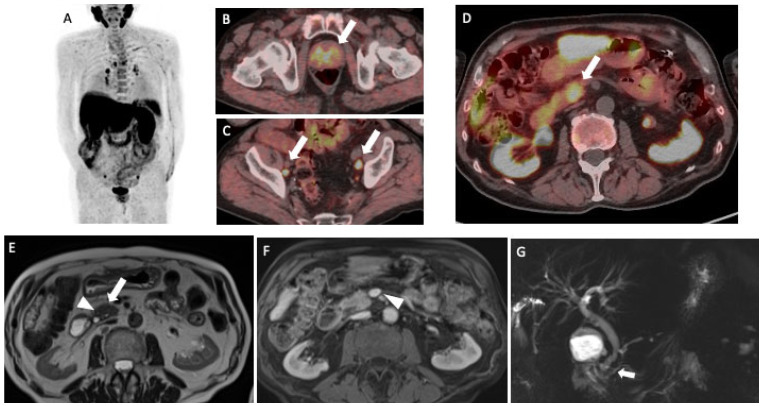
A male patient with prostate cancer underwent 18F-Choline (FCH) PET/CT for the suspicion of lymph node recurrence during hormonal therapy. He was not submitted to local therapies (i.e., surgery or radiotherapy) for the treatment of the primary prostate lesion. The patient has, in addition, a history of diabetes mellitus type II and hypertension. PET/CT detected multiple areas of focal FCH uptakes ((A); maximum intensity projection—MIP) in prostate gland (arrow, (B)) and abdominal-pelvic lymphadenopathies (arrows, (C)), compatible with recurrent prostate cancer. Moreover, a focal tracer uptake was shown in the uncinate process of the pancreas with a maximum standardized uptake value (SUVmax) equal to 13.6 (arrow, (D)). As already stated by Schillaci et al. [1], FCH may physiologically show a moderate-to-high uptake in the liver and the pancreas. However, the presence of a focal uptake should be further investigated in order to make a differential diagnosis with a malignant or benign lesion. A subsequent MRI showed no definite lesion but rather a slight “mass-like” enlargement of the pancreatic head, as visible on the axial single-shot turbo-spin echo T2-weighted image (arrow, (E)). Some micro-cystic areas were appreciated in the pancreaticoduodenal groove (arrowhead, (E)). The absence of lesions was confirmed on the post-contrast pancreatic phase (axial gradient echo volumetric fat saturated image), in which pancreatic parenchyma appears homogeneous, with no solid tissue extending towards major vascular structures (arrowhead, (F)). Of note, the cholangiopancreatography sequence showed a normal-sized but irregular main pancreatic duct, with no cephalic structures (arrow, (G)). Overall, subtle morphological changes and ductal abnormalities supported the diagnosis of CMFP. The Carbohydrate antigen 19–9 (CA19.9) was normal (17.0 UI/mL). However, after one month from MRI and FCH PET/CT, the patient was submitted to an endoscopic ultrasound (EUS) exam and biopsy, based on the suggestion of the multidisciplinary team.
